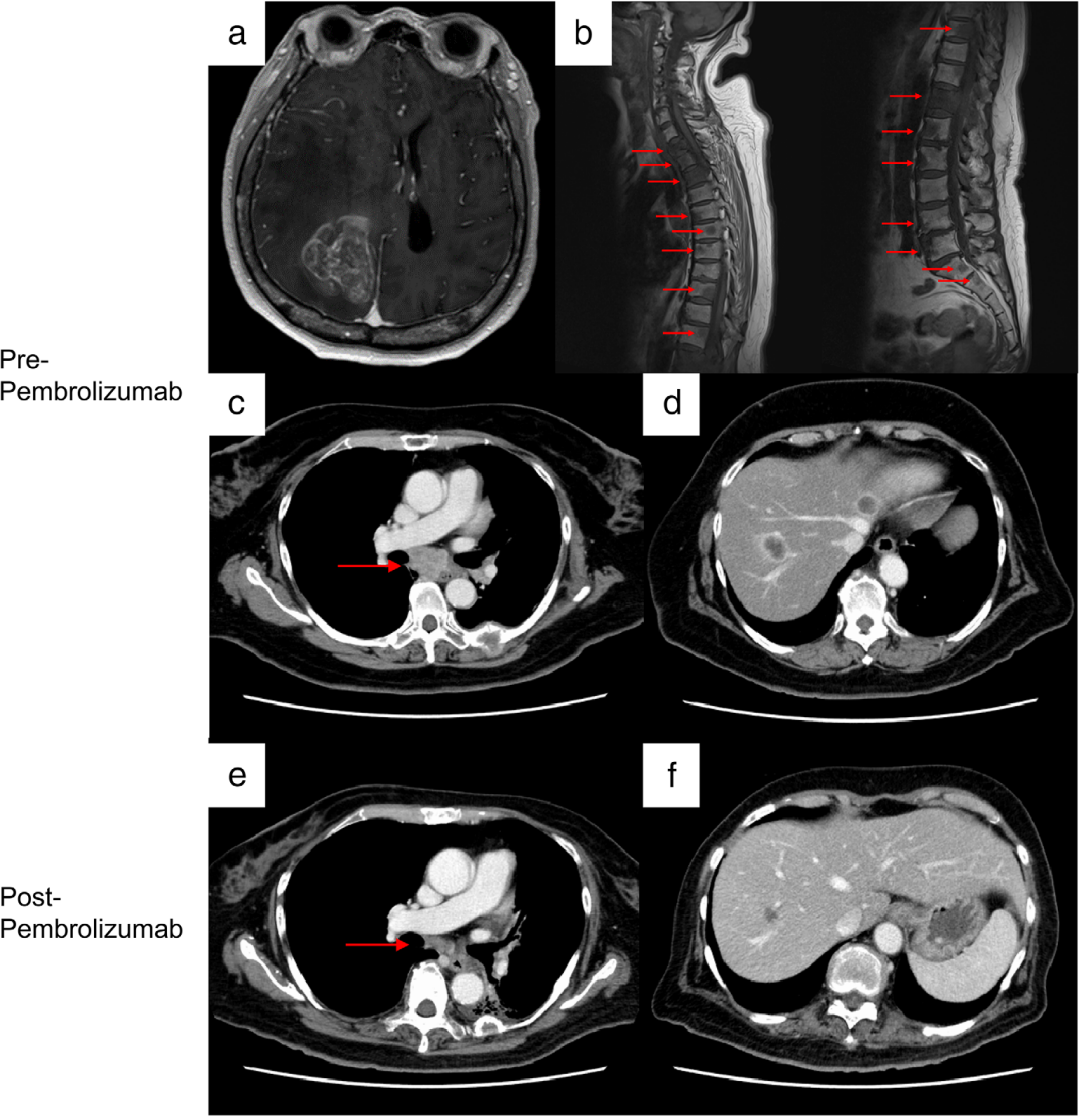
Brain and spinal cord MRI (contrast‐enhanced, T1‐weighted imaging) before pembrolizumab treatment showed (a) intratumoral bleeding from the brain metastasis in the right occipital lobe and (b) multiple bone metastases (red arrows). Chest and abdominal CT showed (c and e) mediastinal lymph node metastases (red arrows) and (d and f) liver metastases (red arrows) before and after pembrolizumab treatment.
